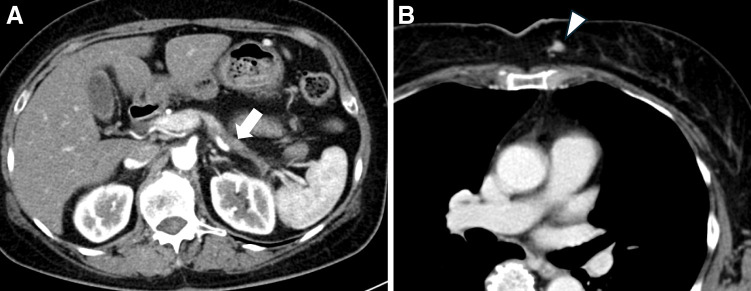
CT images. (A) Abdominal contrast-enhanced CT at 3 months after treatment initiation showed marked reduction of the pancreatic tail tumor (white arrow), with liver metastases undetectable on imaging. (B) The breast mass also showed a reduction in size (white arrowhead).
